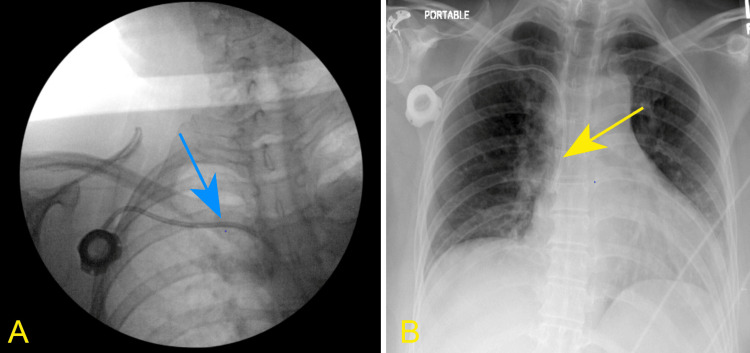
(A) Fluoroscopic placement of the intracardiac MediPort (blue arrow). (B) Chest X-ray demonstrating well-placed intracardiac tip of the MediPort (yellow arrow).
